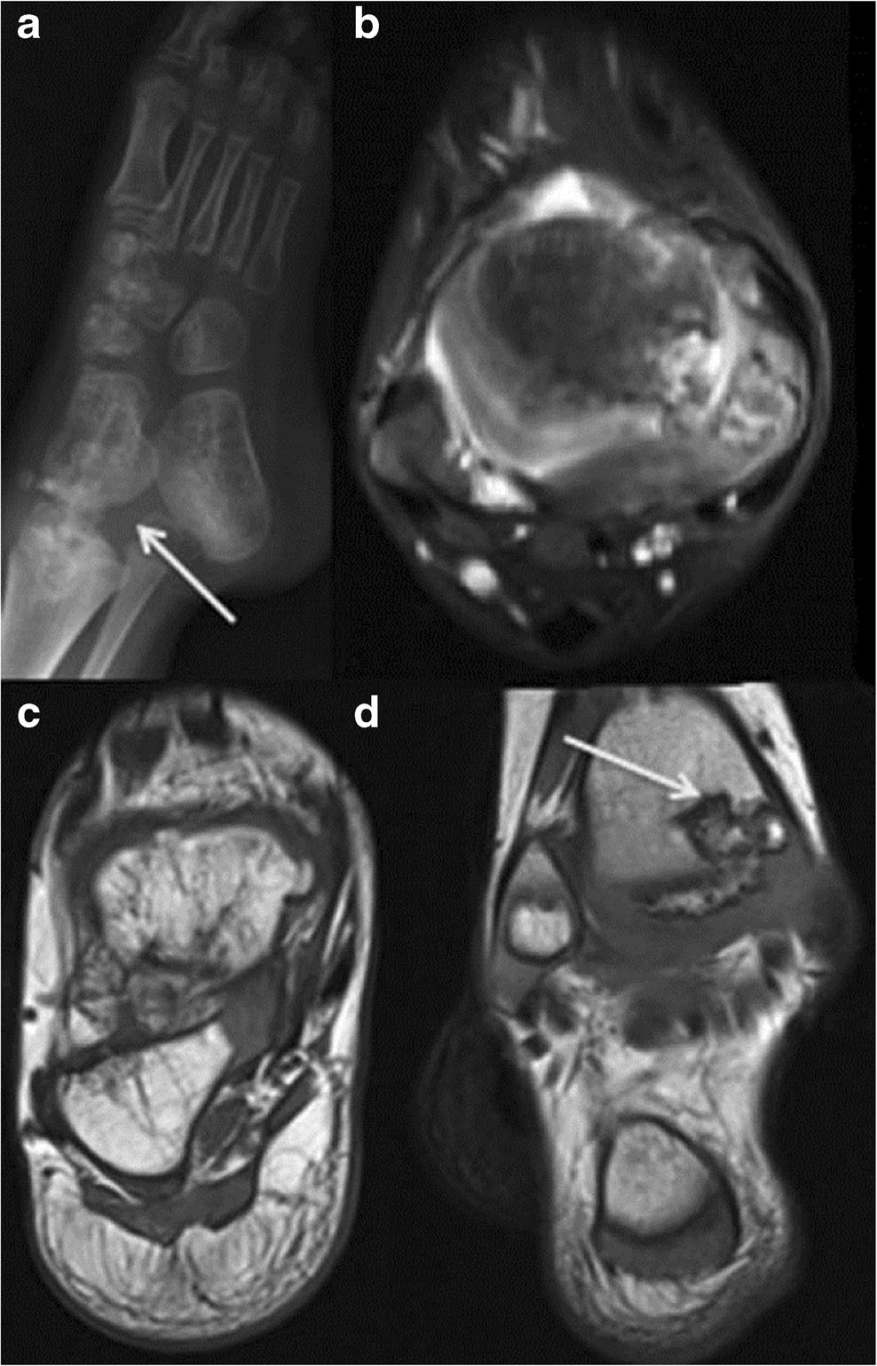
Trevor’s disease (dysplasia epiphysealis hemimelica) in 3-year-old boy. Antero-posterior radiograph of the left foot a shows asymmetric osteochondral overgrowth arising from the medial epiphysis of the tibia (*arrow*) and from multiple ossification centers within the tarsal bones of the foot. An axial STIR image b and axial and coronal T1 images c and d demonstrate cortico-medullary coalescence and cartilaginous signal (*arrow*)Heterotopic ossificationThese represent localized, non-neoplastic, reparative lesions in soft tissues separate or adjacent to bone, which are comprised of reactive hypercellular fibrous tissue, cartilage, and/or bone. They can arise secondary to trauma (*myositis ossificans circumscripta*, *ossifying hematoma*), or without a history of prior injury.On MR, variable low signals on T1WI, PDWI, and T2WI are noted, depending on the degree of mineralization/ossification, fibrosis, and hemosiderin deposition (Fig. 9). Zones of high signal on T1WI and T2WI may occur from fatty marrow metaplasia. Enhancement with gadolinium contrast or associated abnormal marrow signal are usually absent [2]
